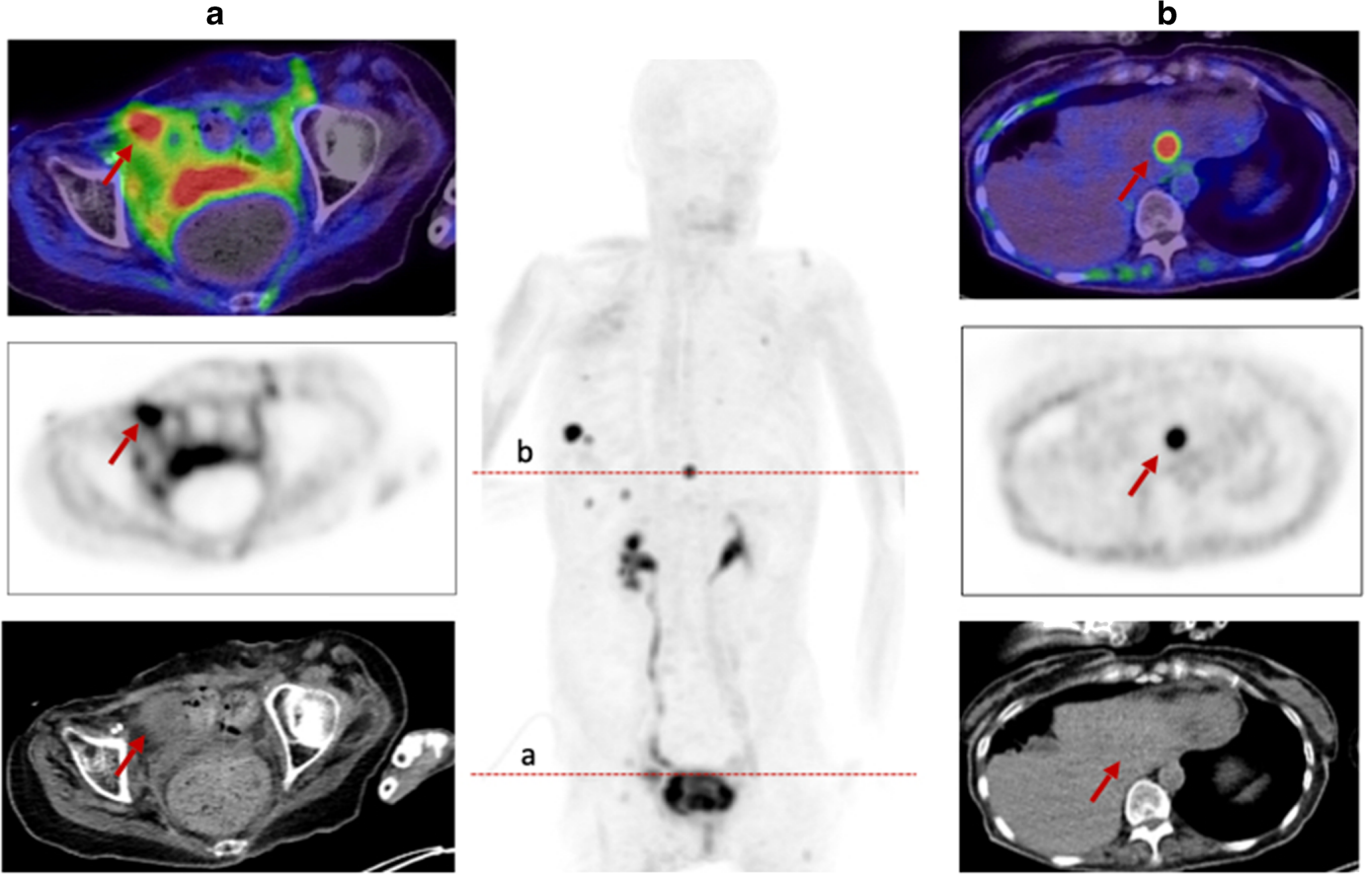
A 60-year-old patient with metastasized cervical carcinoma underwent ^68^Ga-FAPI-PET/CT due to monitoring. The local relapse presented a rather strong FAPI uptake with SUVmax 16.90, similar to the exemplary shown liver metastasis in segment II (SUVmax 14.1)
